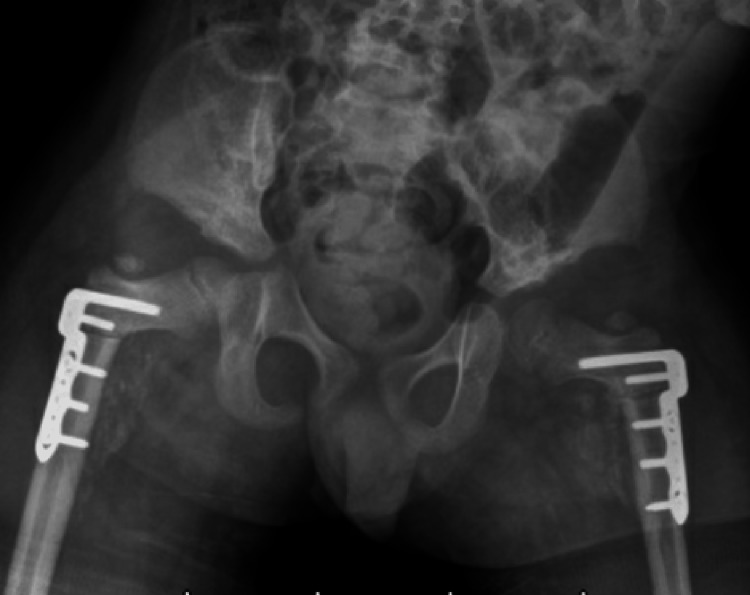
AP radiograph of the pelvis at 3 weeks follow up demonstrating bilateral well reduced hips with implants in place. There is early callus formation at the osteotomy sites.
